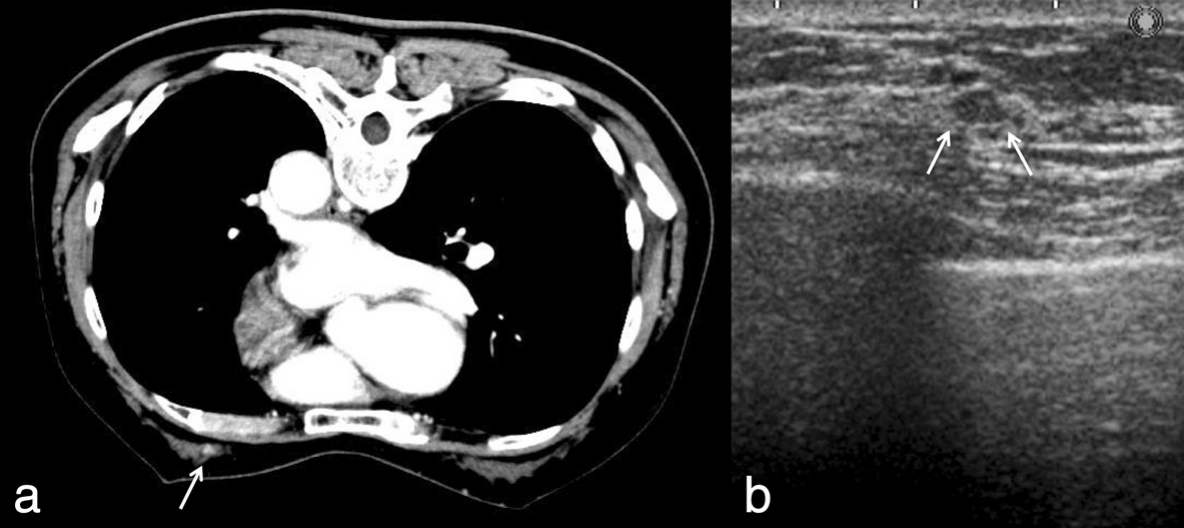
MDCT showed enhanced focus on the contralateral breast. Targeted sonography showed a hypoechoic mass connecting to the adjacent ducts, the size of which was 5x2 mm. Sonography-guided vacuum-assisted core needle biopsy was performed to obtain a definitive diagnosis. The pathological diagnosis was hyperplasia.
